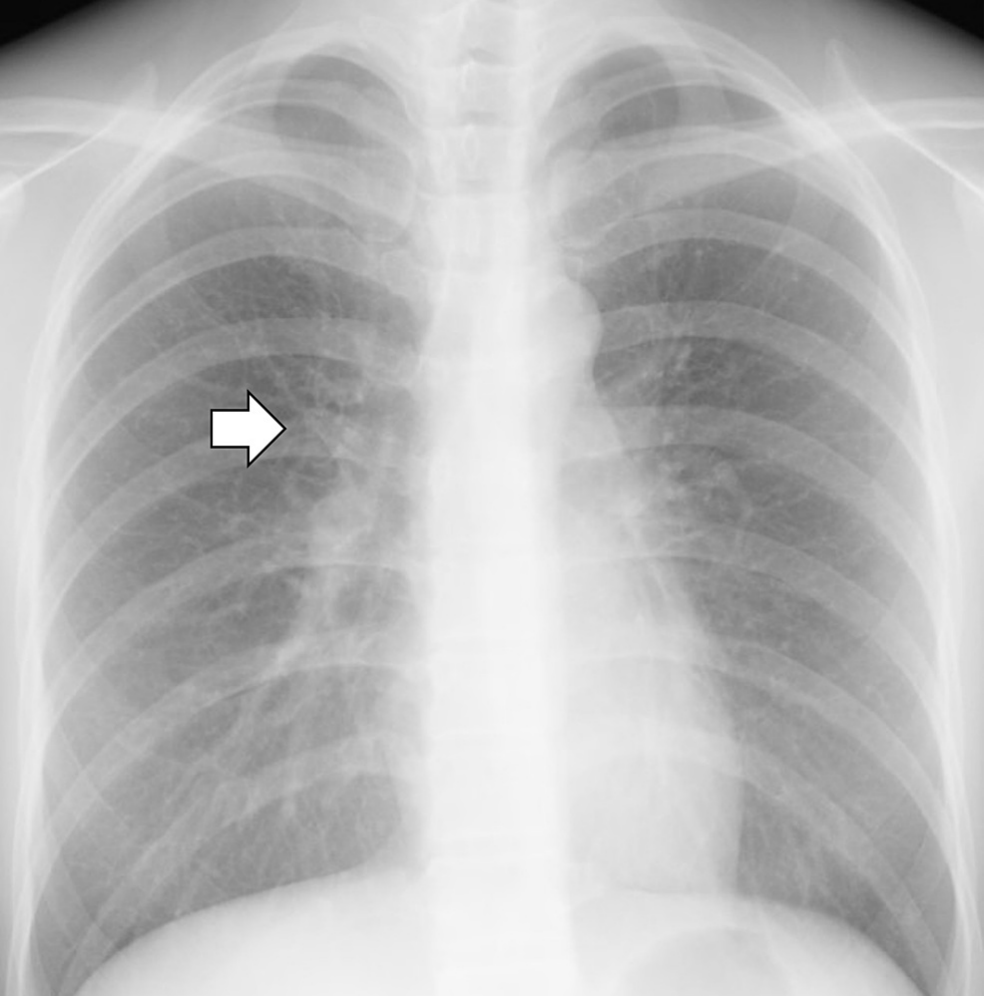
Chest radiography revealing a remarkable improvement of bilateral hilar enlargement
